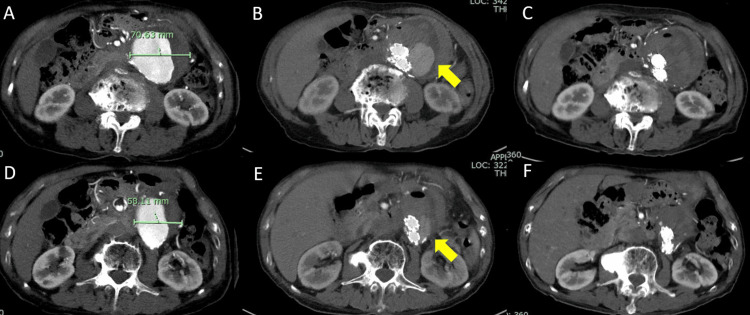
Contrast-enhanced computed tomography findings (A, D) Preoperative imaging. (B, E) Images obtained on day 5 after surgery. (C, F) Images obtained on day 12 after surgery. Preoperative contrast-enhanced computed tomography revealed large abdominal aortic aneurysm (A: same level of B and C, D: same level of E and F). Five days after surgery, a large amount of contrast medium leakage was observed in the aneurysm (arrows, B, E). The contrast medium in the aneurysm had disappeared 12 days after the operation (C, F).
